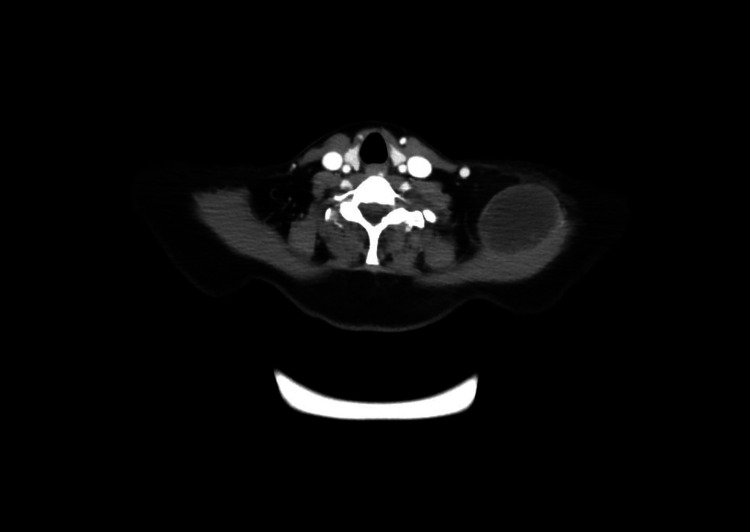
CT of chest/abdomen/pelvis showing progressive disease with a 4.4 x 5.5 cm left trapezius mass
